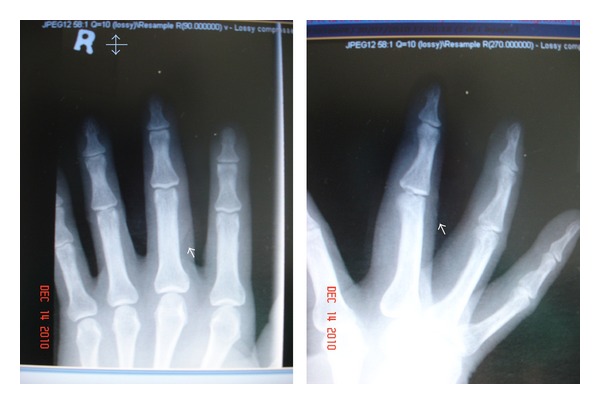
Anteroposterior and lateral views of plain radiographs of the hand. There is no evidence of bony irregularity.
